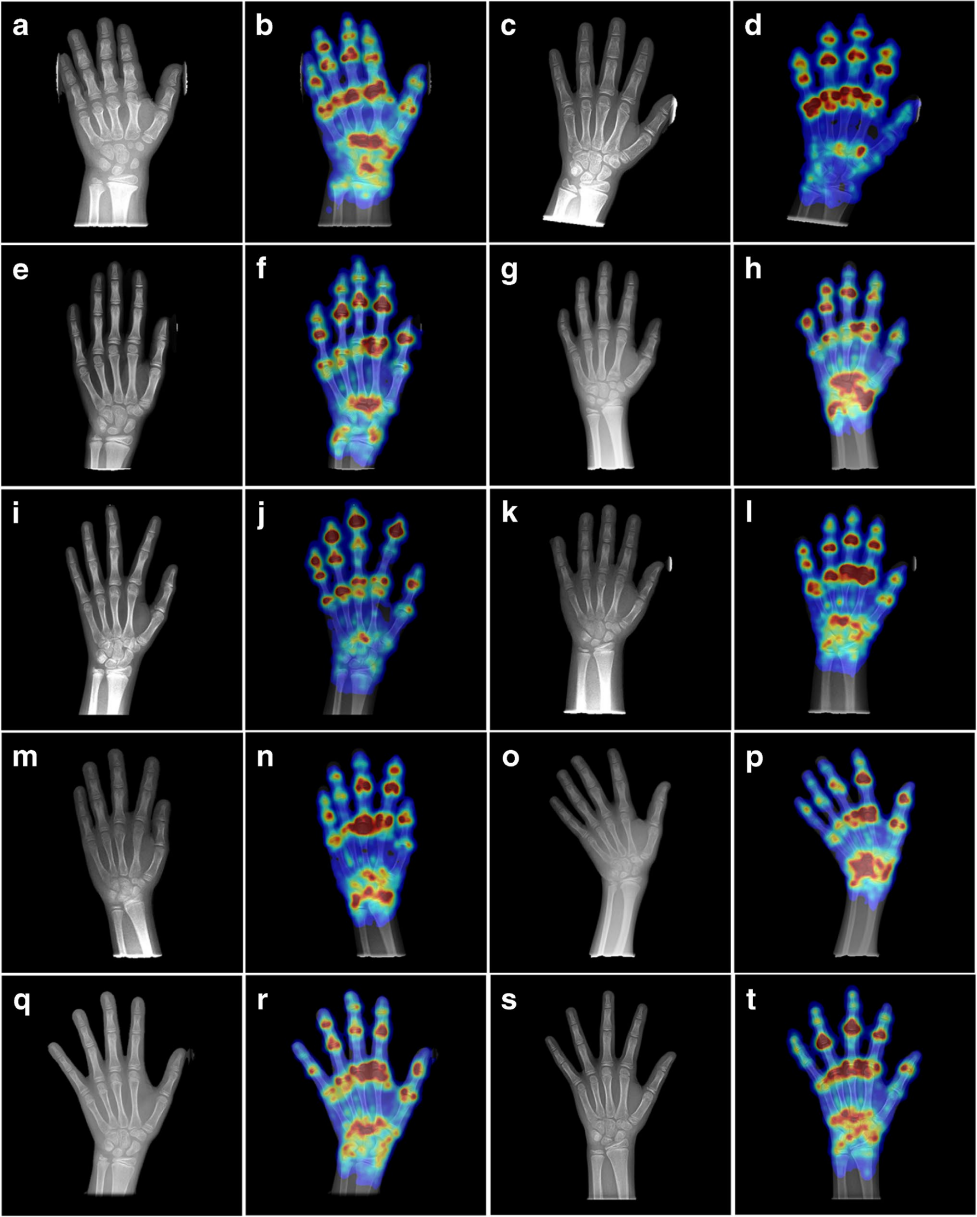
Example attention maps from Deeplasia. a, b A 7-year-old girl with achondroplasia. c, d A 10-year-old girl with hypochondroplasia. e, f A 10-year-old girl with intrauterine growth restriction. g, h A 10-year-old girl with Noonan syndrome. i, j An 11-year-old girl with pseudohypoparathyroidism. k, l A 10-year-old girl with a mutation of the *SHOX* gene. m, n A 10-year-old girl with Silver-Russell syndrome. o, p A 9-year-old girl with Ullrich-Turner syndrome. q, r A 9-year-old girl with no genetic disorder. s, t A 9-year-old girl with no genetic disorder. For each example, the radiograph (a, c, e, g, i, k, m, o, q, s) and the attention map (b, d, f, h, j, l, n, p, r, t) is shown. These maps show that the attention of the models is mainly on the phalangeal and metacarpal joints, as well as the carpal bones, i.e. the regions relevant for bone age assessment. Larger versions of these images, as well as the exact bone age measurements, are available in Supplementary Material 4
